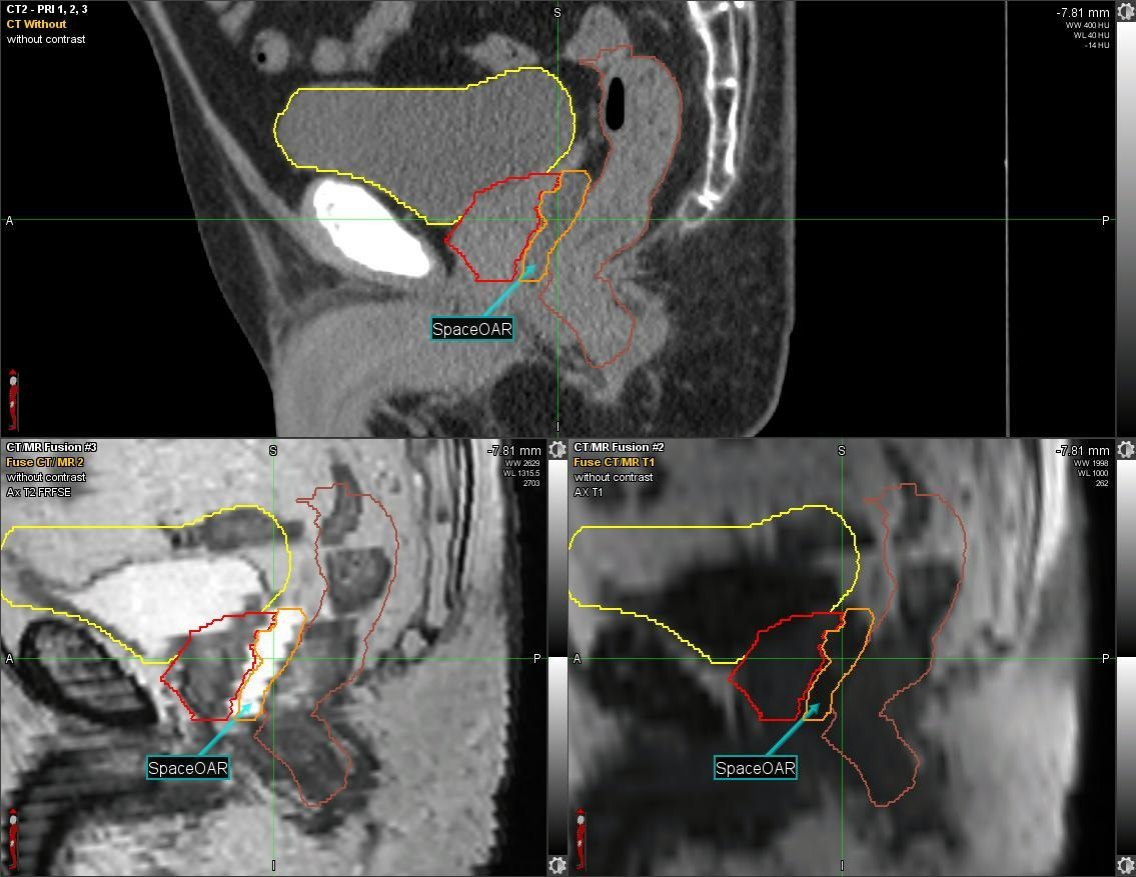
CT and MRI: Sagittal View Yellow = Bladder Red = Prostate Orange and Arrow = Spacer Brown = Rectum Computerized tomography: CT Magnetic resonance imaging: MRI
